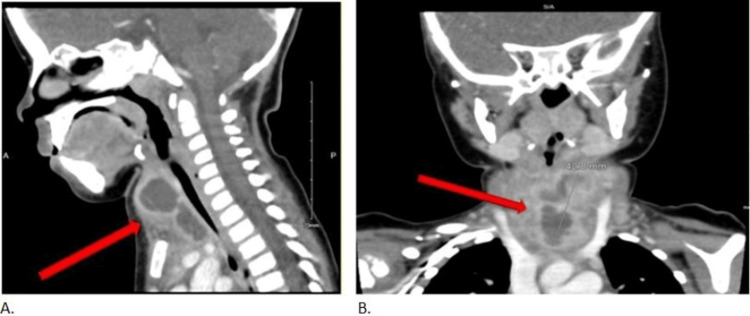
(A) Sagittal view and (B) coronal view CT of the neck The images show an anterior midline neck collection that is multi-septated with thick enhancing irregular walls extending to the superior mediastinum, with no evidence of significant airway narrowing.
